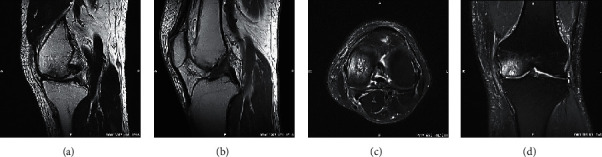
MRI scan images: (a) sagittal T1WI, (b) sagittal T2WI, (c) cross-sectional SE-T2WI, and (d) coronal SE-T2WI.
